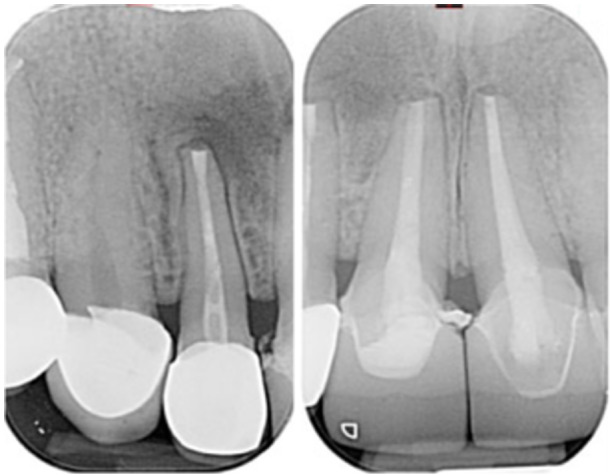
Case 3: The preoperative CBCT and periapical radiograph show an extensive periapical radiolucency involving teeth 11 and 12, with a loss of the labial cortical bone. Apical surgery was initially performed on teeth 11 and 12, but a few months later, tooth 21 required RCT and apical surgery (case 7). At 6 months, marked healing can be seen, evidenced by a reduction in the periapical radiolucency size associated with teeth 11 and 12. Note that the crowns on teeth 11 and 21 have also been replaced with new ones. Cases 3 and 4 are from the same patient but with different L‐PRF preparations.
